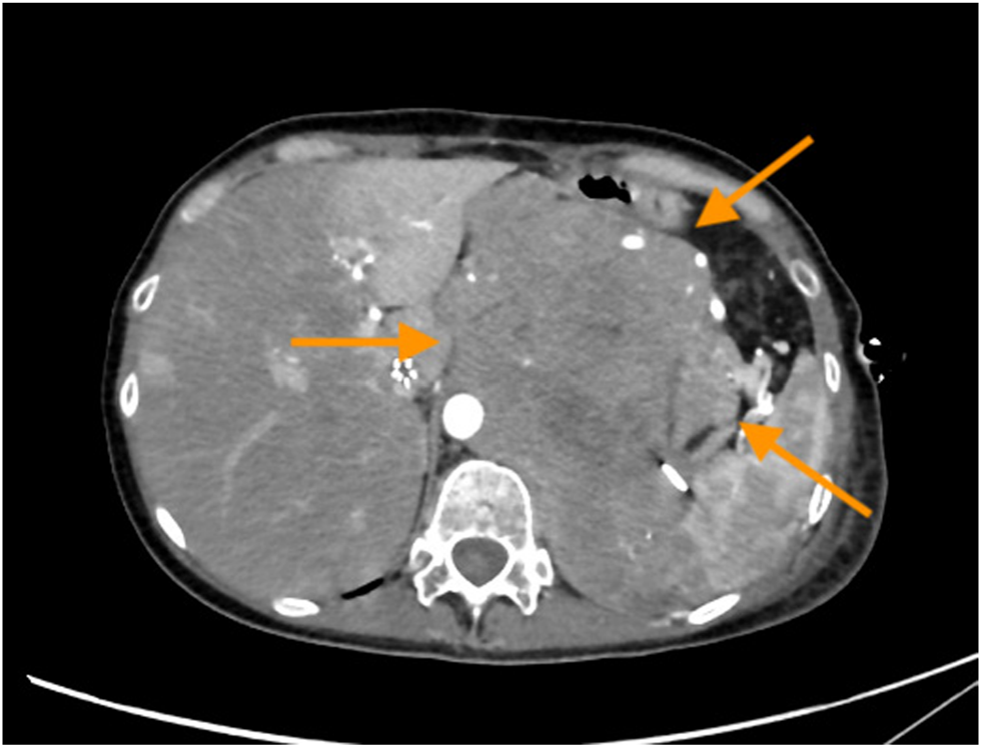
CT-scan, the imaging revealed a huge recurrence in the surgical site (arrows) with involvement of thoracic wall.
